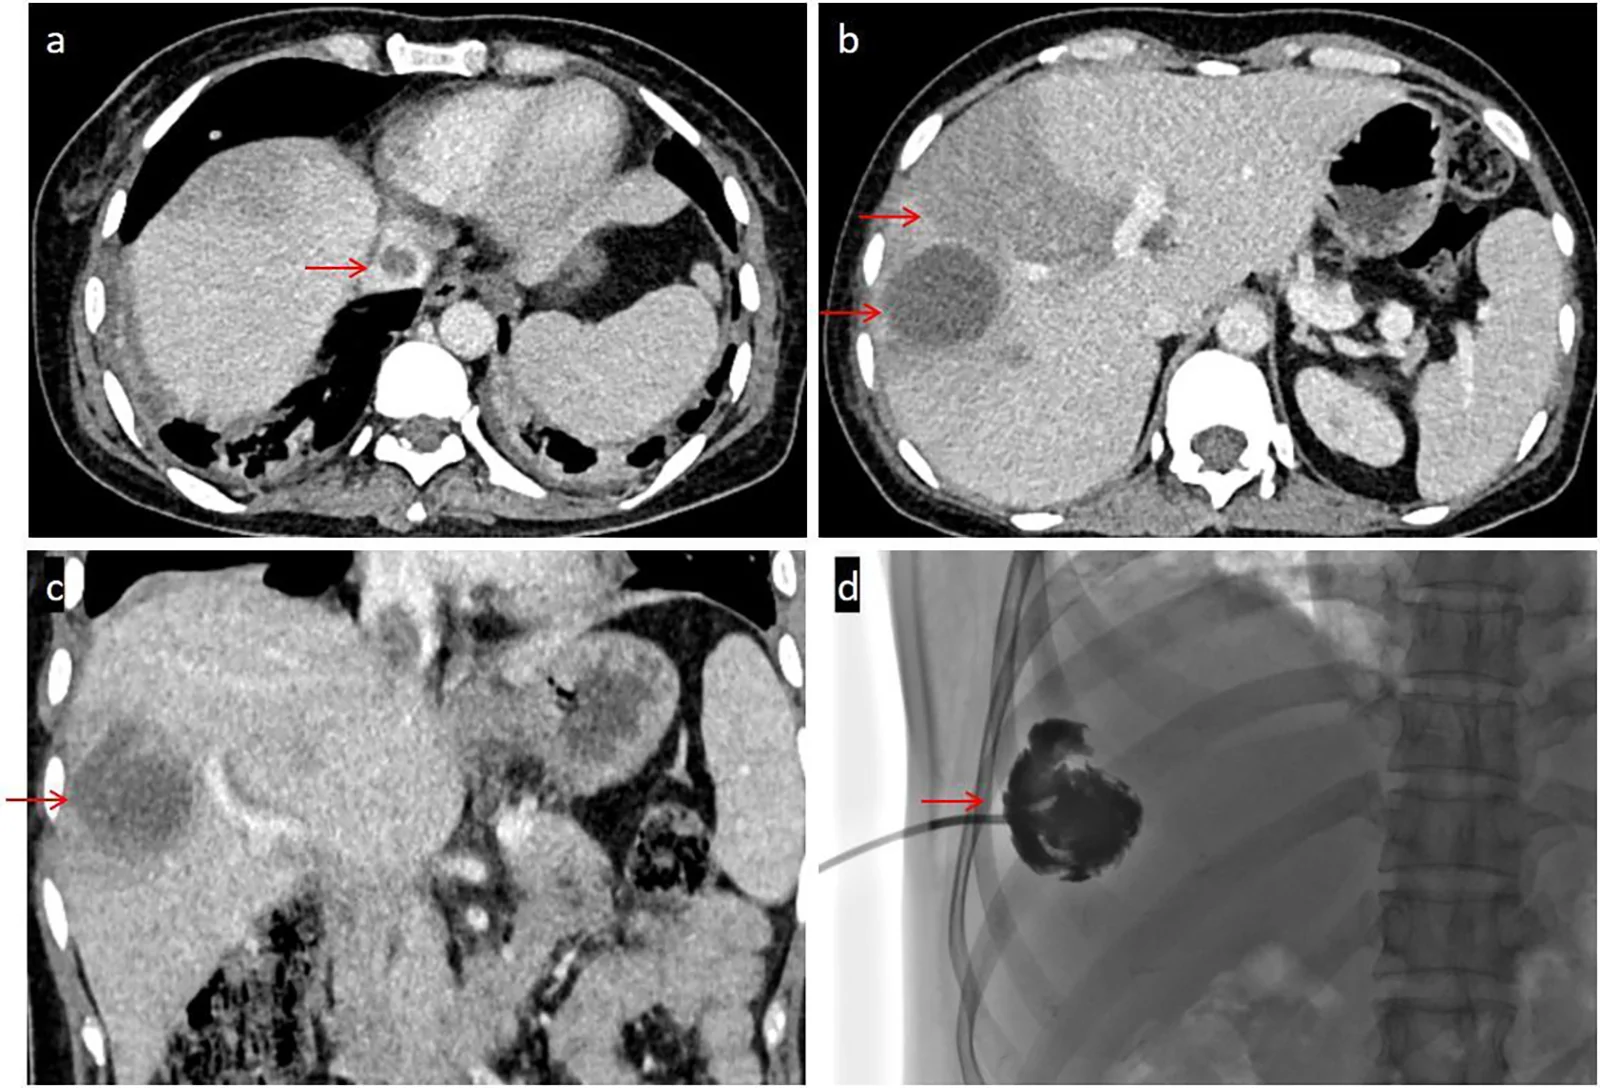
Upper abdominal contrast-enhanced CT and percutaneous liver abscess puncture drainage. (a) Revealed a bacterial embolus in the retrohepatic IVC. (b,c) Showed thrombosis in the right and middle hepatic veins, accompanied by hepatic ischemia and infarction in the drainage territory of the middle hepatic vein; additionally, a liver abscess was also identified in the corresponding hepatic region. (d) Showed the performance of percutaneous liver abscess puncture drainage.
